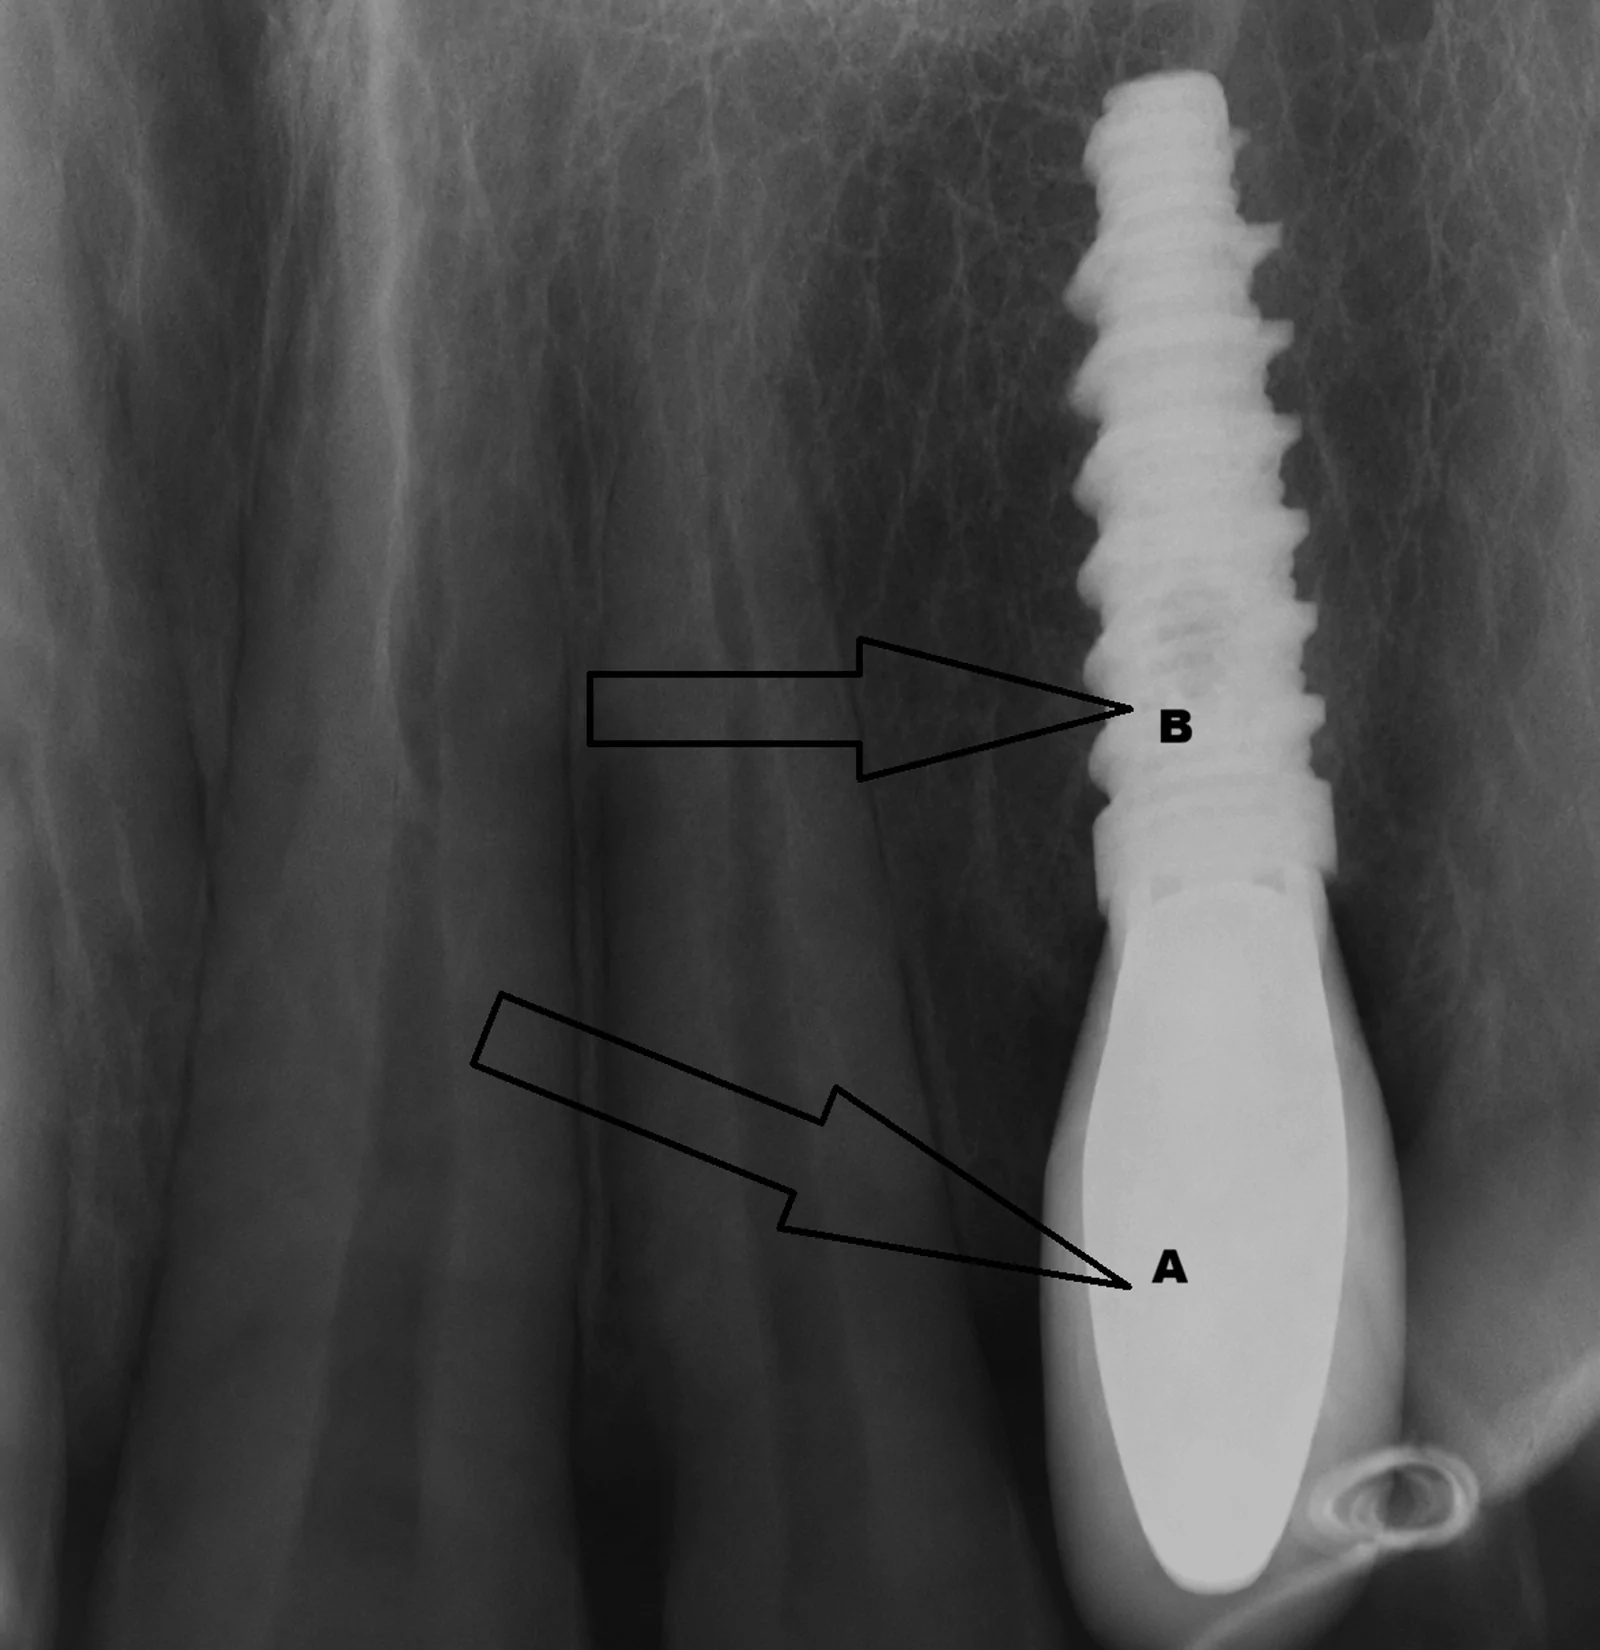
Definitive Implant-Supported Restoration Following Osseointegration. Postoperative periapical radiograph demonstrating the definitive implant-supported restoration after successful osseointegration. The lower black arrow (A) indicates the definitive implant-supported crown, while the upper black arrow (B) indicates the dental implant, demonstrating satisfactory positioning and favorable peri-implant bone support at the time of restoration delivery.
